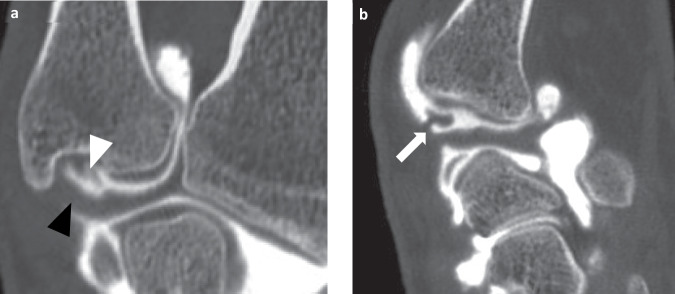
Arthro-CT of a 33-year-old man with ulnar-sided wrist pain and no radioulnar instability; no lesions were described in the initial report. a Coronal view of the TFCC showing an isolated tear of the foveal bundle (white arrowhead) with an intact styloid bundle (black arrowhead). b Sagittal view demonstrating dorsal extension of the tear into the DRUL (white arrow)
